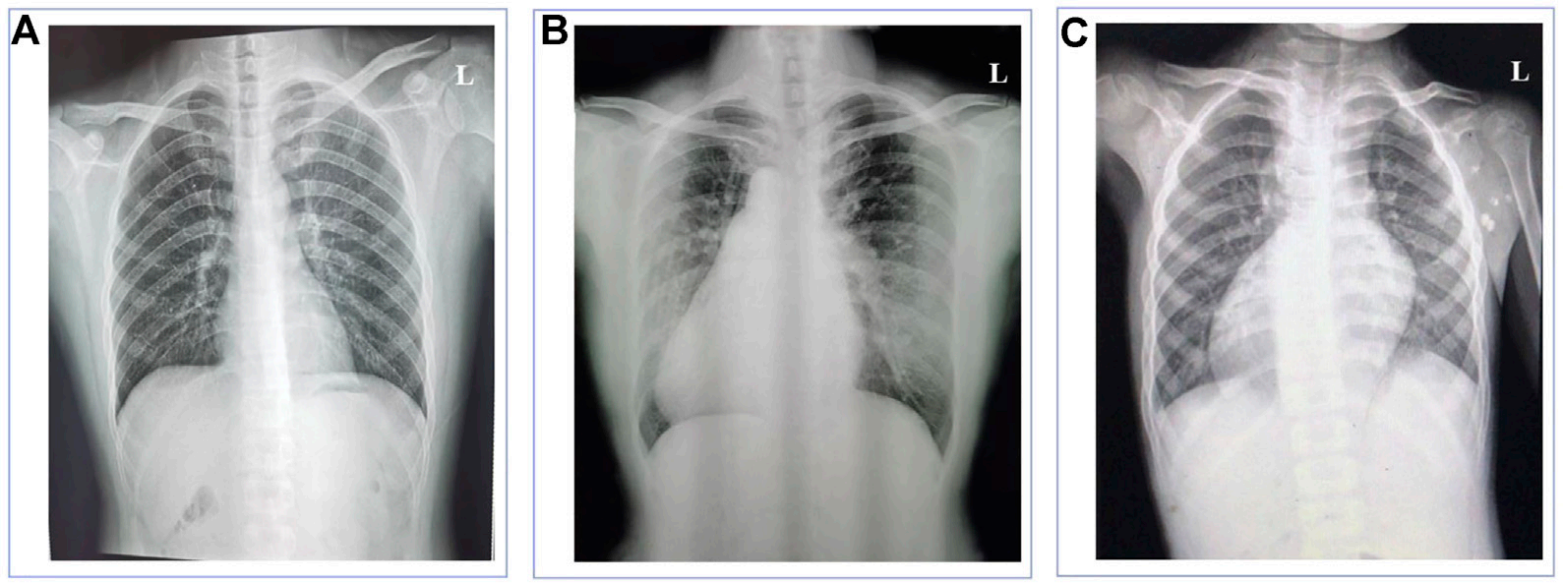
Chest X-ray images of the normal individual and patients with left-right asymmetry disorders. (A) Chest X-ray of the normal individual presented normal organ placement. (B) Chest X-ray of the proband in family 1 revealed dextrocardia. (C) Chest X-ray of the proband in family 2 revealed dextrocardia.
